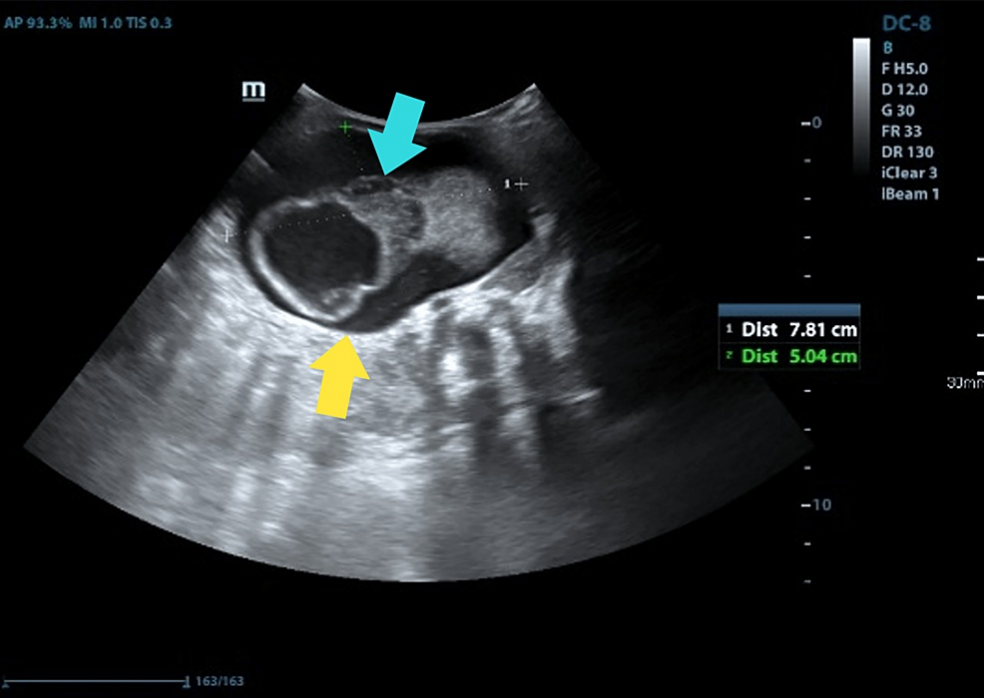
Abdominopelvic USG of the newborn demonstrating a well-defined cystic structure seen in the center of the abdomen to the left side measuring about 80 x 50 millimeters with heterogeneous soft tissues content which is highly suggestive of a nonviable fetus (blue arrow) seen inside the cyst (yellow arrow). Empty skull with vertebral organization and limb buds formation were also visualized. USG: ultrasonography
